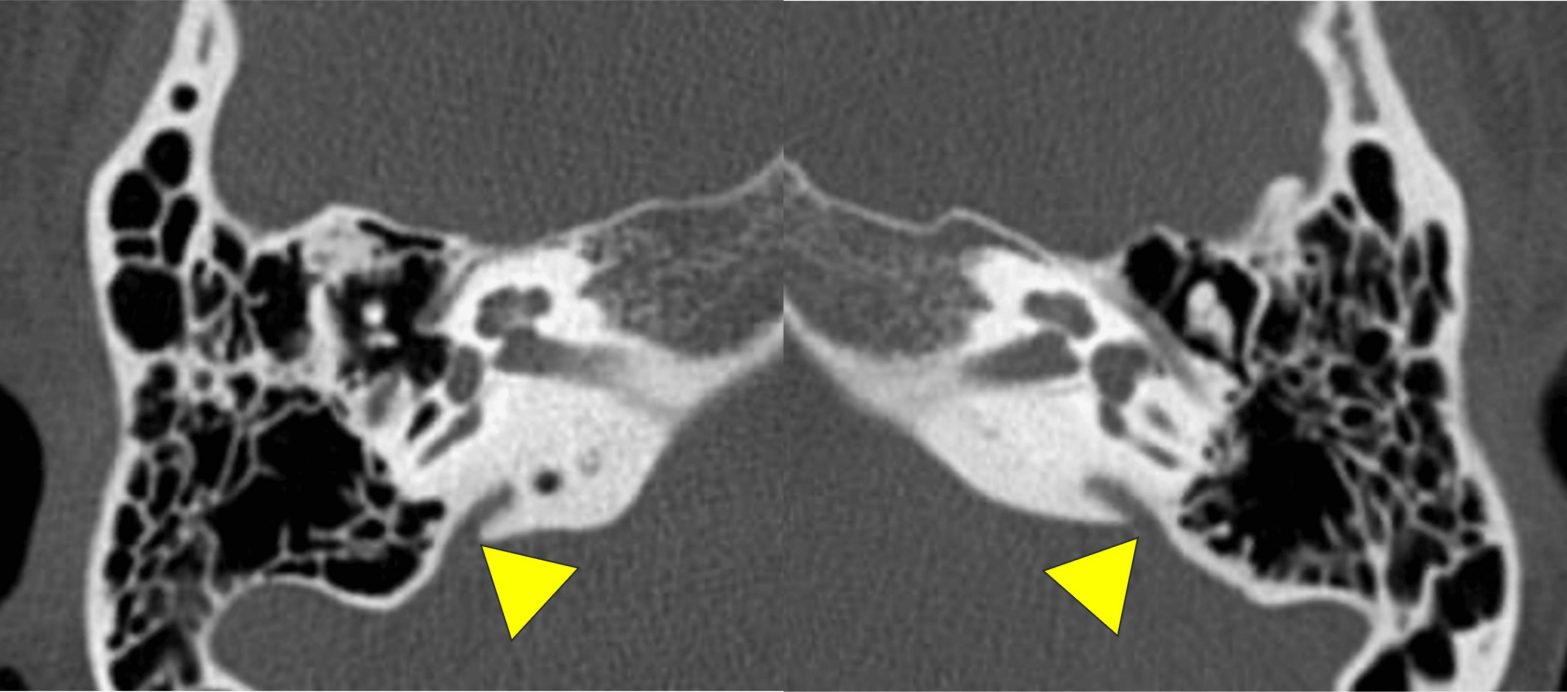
Pre-operation HRCT of the temporal bone (axial plane). Arrowheads indicate an enlarged vestibular aqueduct at the operculum. HRCT: High-Resolution Computed Tomography.
